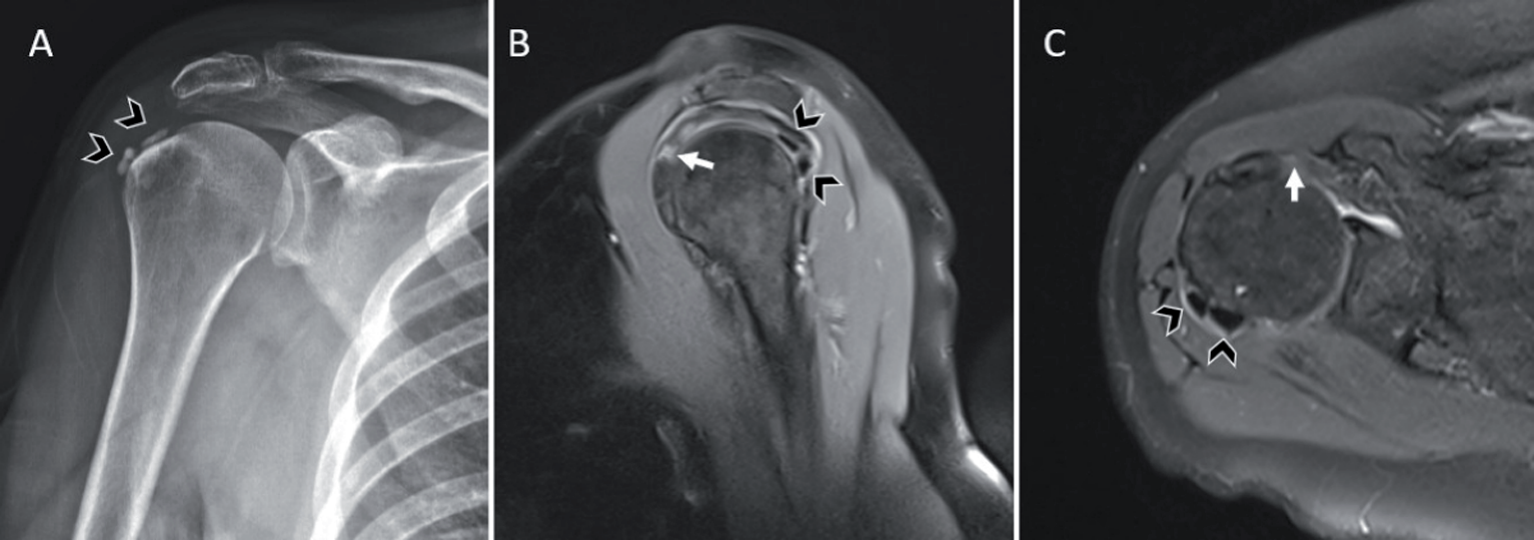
Calcific tendinitis associated with a tear in a different tendon. Right shoulder images of a 51-year-old female patient.(A)Plain radiograph of the right shoulder demonstrates 2 calcific radiopaque foci adjacent to the humerus, the largest measuring approximately 10 mm in diameter (arrowheads).(B)Sagittal proton density (PD) and(C)axial PD-weighted magnetic resonance imaging images show 2 calcific deposits within the infraspinatus tendon, the largest measuring approximately 10 mm (arrowheads). In the same images, an articular-sided partial-thickness tear of the subscapularis tendon is identified (arrow).
